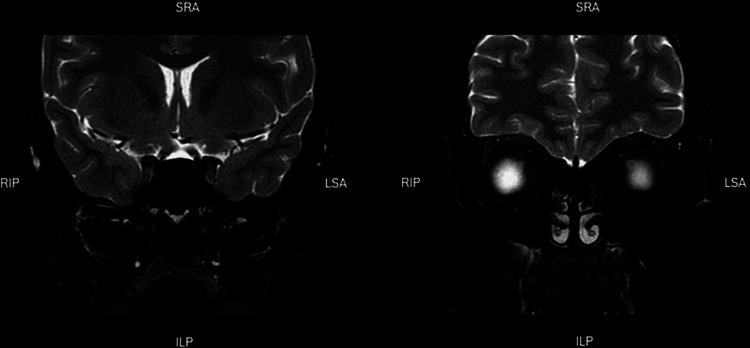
Coronal T2W MRI of the brain that was repeated after three weeks after hospital discharge. The optic nerves appeared of normal caliber. There was a stable subtle asymmetrical enhancement of the prechiasmatic segment of the left optic nerve. No other significant enhancement of the optic pathway noted.
